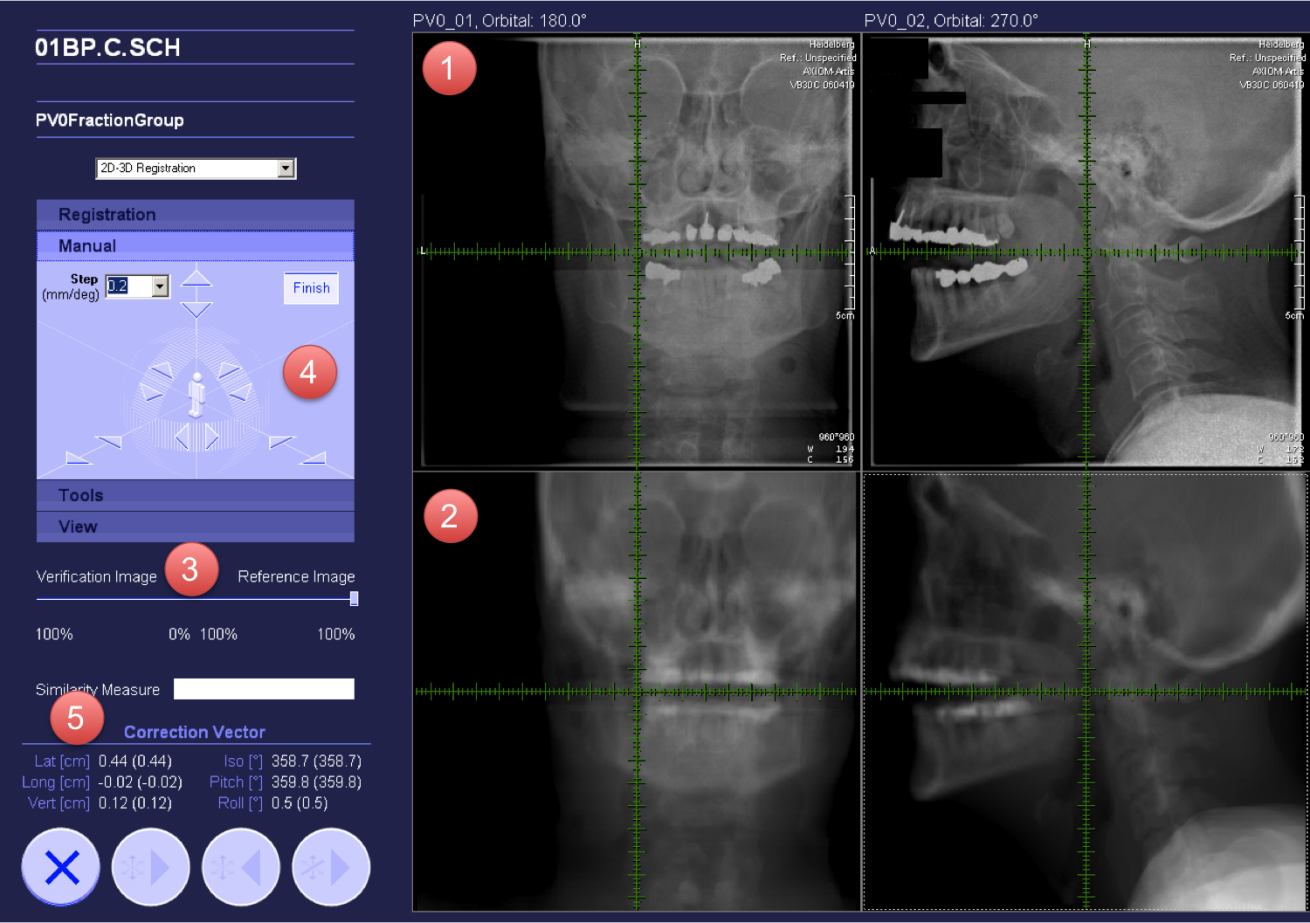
Particle therapy position correction tool: 1: orthogonal x-rays acquired for position control at each session 2: digitally reconstructed radiographs (DRRs) from the planning CT-scan 3: slider to switch between the x-ray (position control) and DRR (planning scan). 4: manual correction tool for 6 DOF 5: resulting correction vector.
